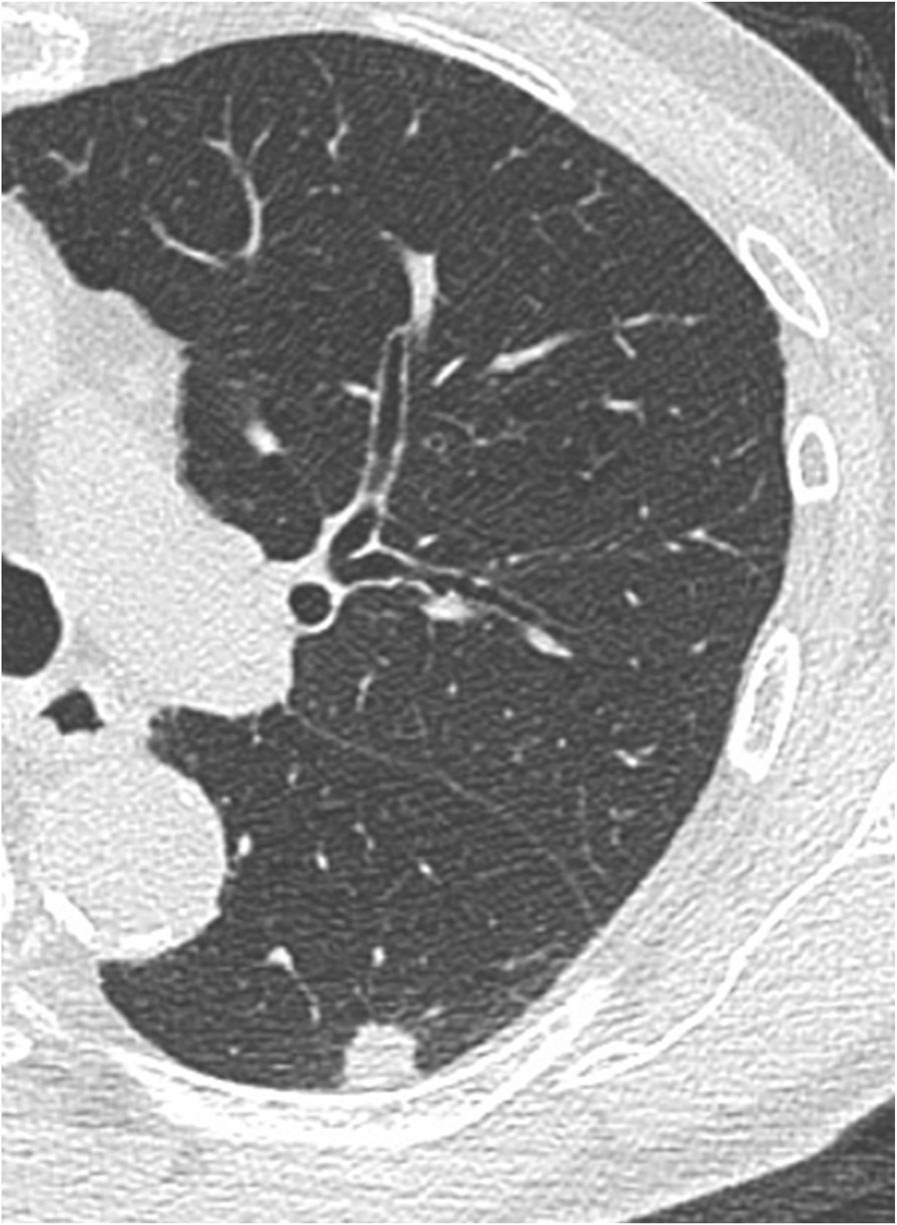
Chest computed tomography image showing a solid nodule in the left lower lobe
